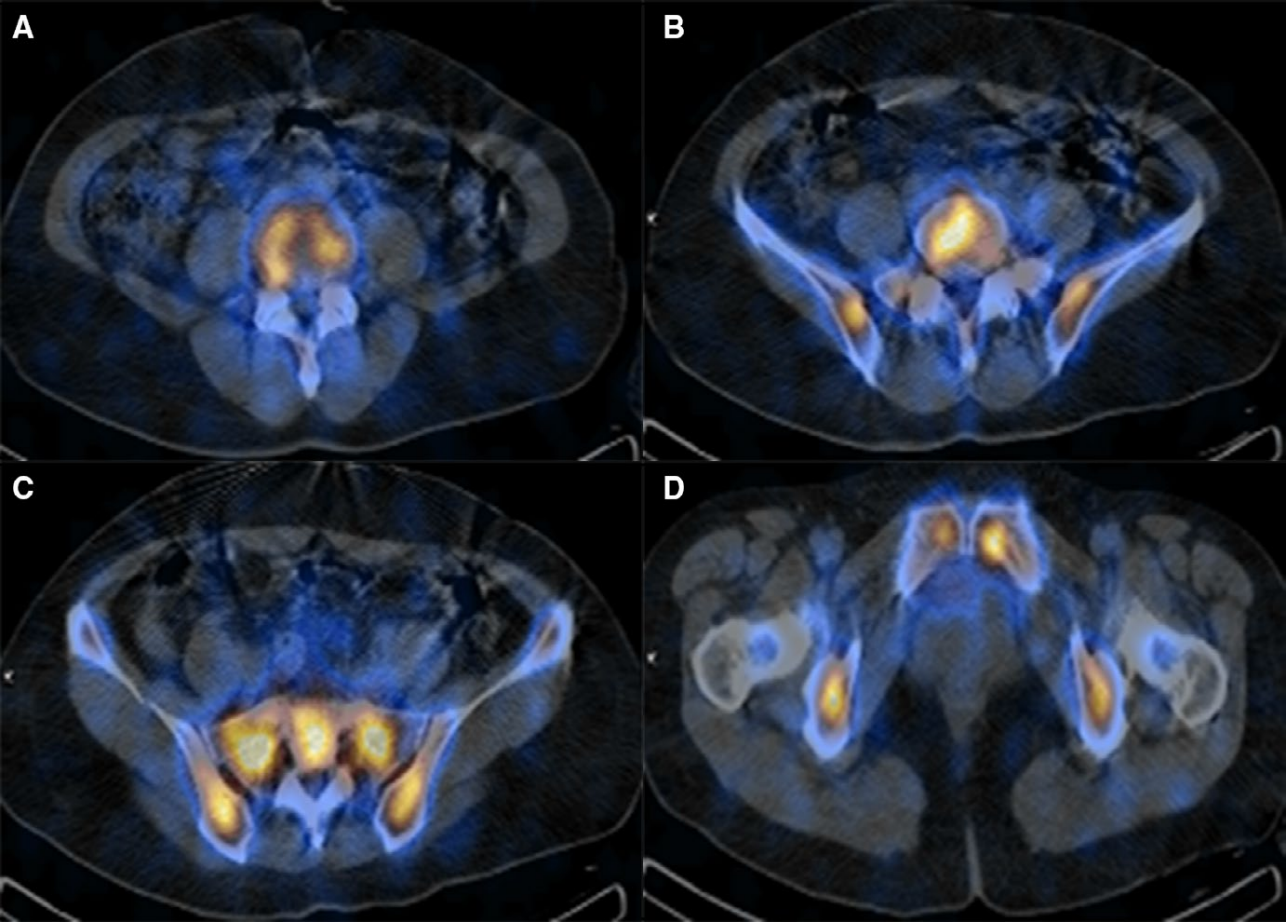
The representative image of the SPECT images fused into CT images. A, L4 vertebral bodies; B, L5 vertebral bodies and iliac crests; C, sacrum and iliac crests; D, pubic symphysis
